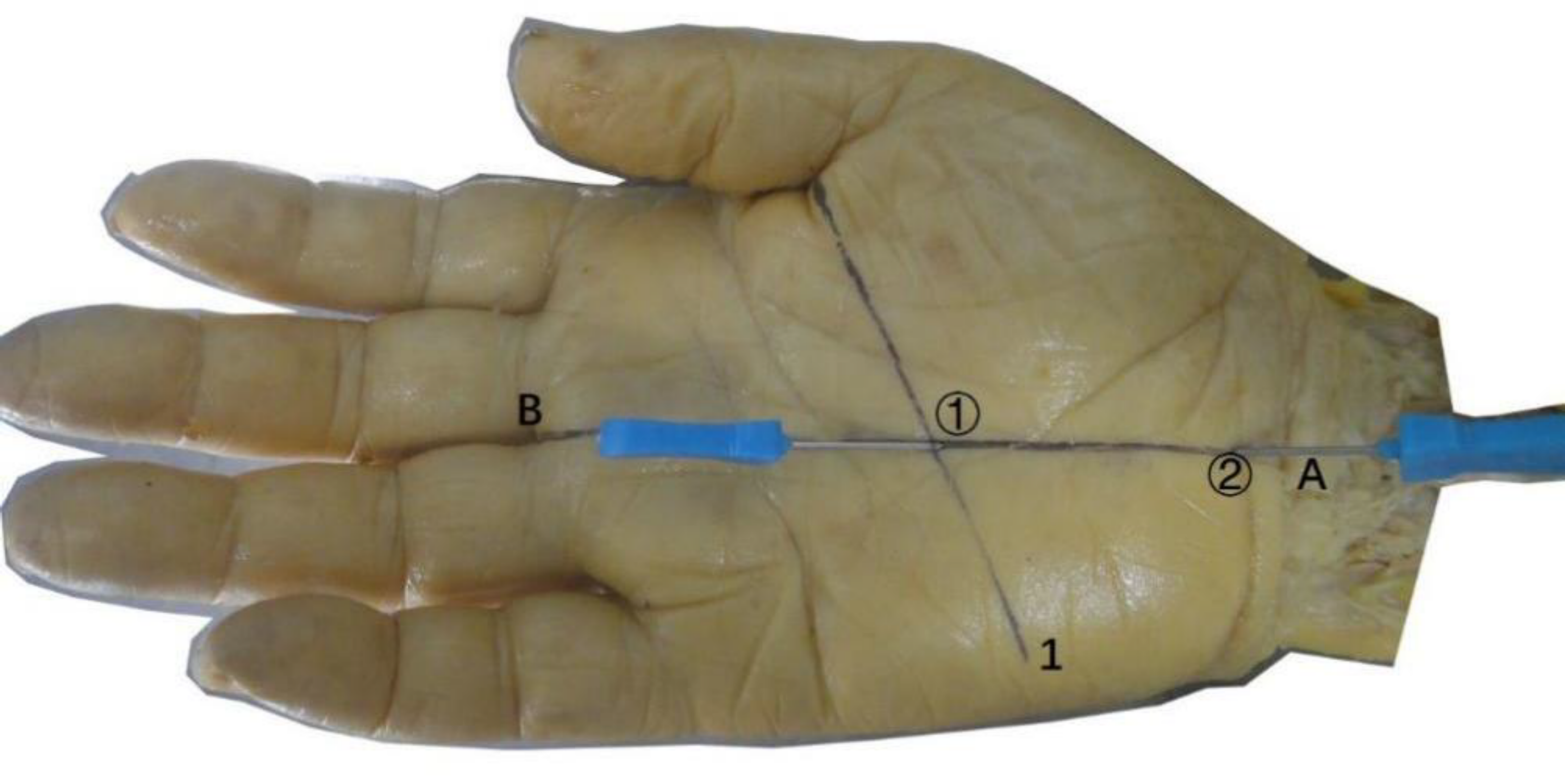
The completed needle insertion technique of the non-ultrasonic guided needle knife release technique. ①, needle entry point 1; ②, needle entry point 2; 1, Kaplan line; (A) the intersection point of the palmar longus tendon with 3 mm lateral to the ulnar side and the transverse stripes of the proximal wrist; (B) the intersection of the ring finger and the middle finger.
